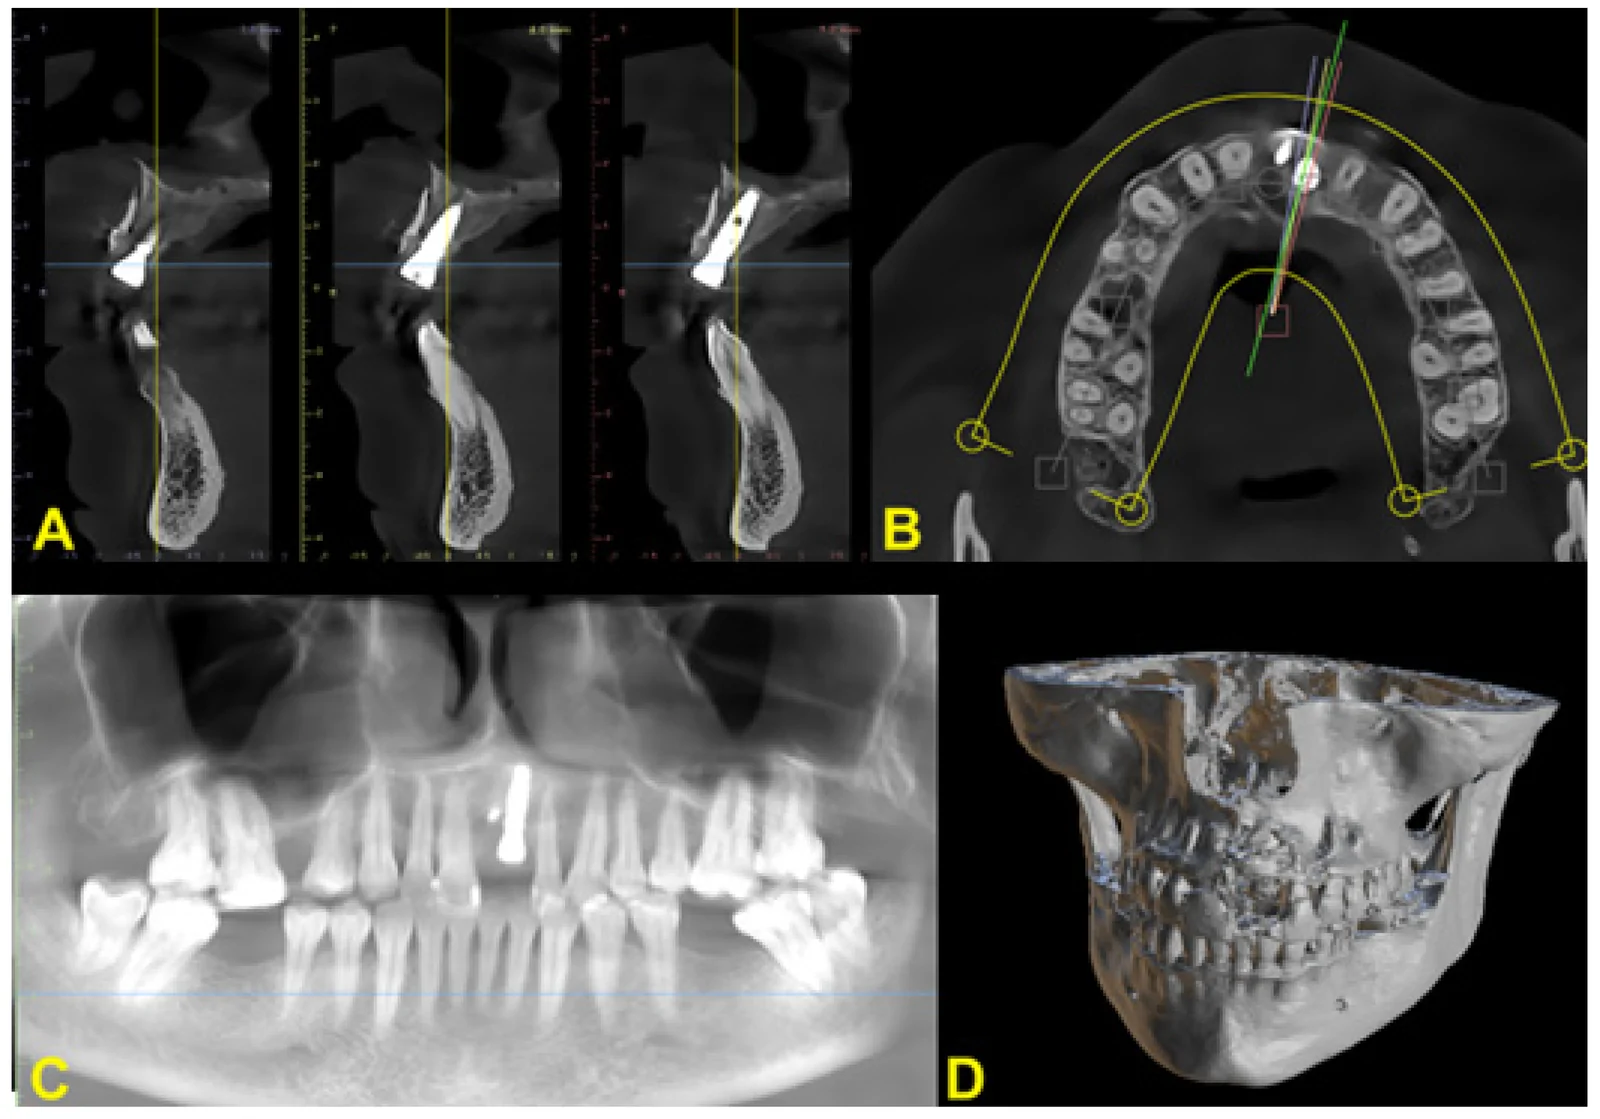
Cone-beam computed tomography of tooth 21 position after second implantation: (A) cross-sectional views; (B) axial view; (C) orthopantomographic view; (D) pseudo-3D reconstruction.
